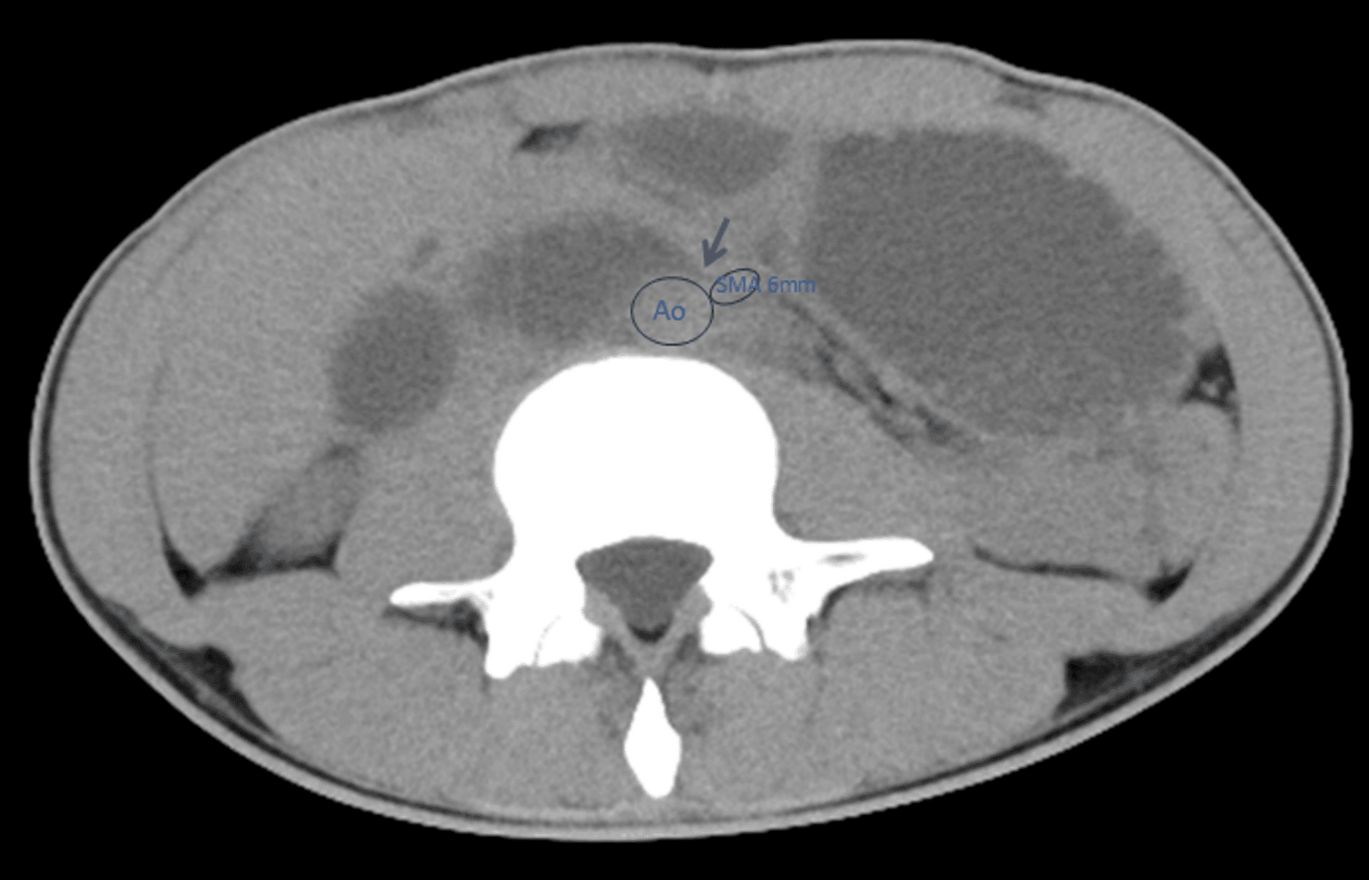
Abdominal computed tomography A bird-beak sign (arrow) was observed at the duodenum, compressed between the aorta and the SMA, accompanied by proximal gastric dilatation. The aortomesenteric distance was 6 mm (≤8 mm), which is the established diagnostic criterion for SMA syndrome. Contrast-enhanced CT was not performed; therefore, the aortomesenteric angle (≤25°), which is also the diagnostic criterion for SMA syndrome, could not be evaluated. Ao, aorta; SMA, superior mesenteric artery.
